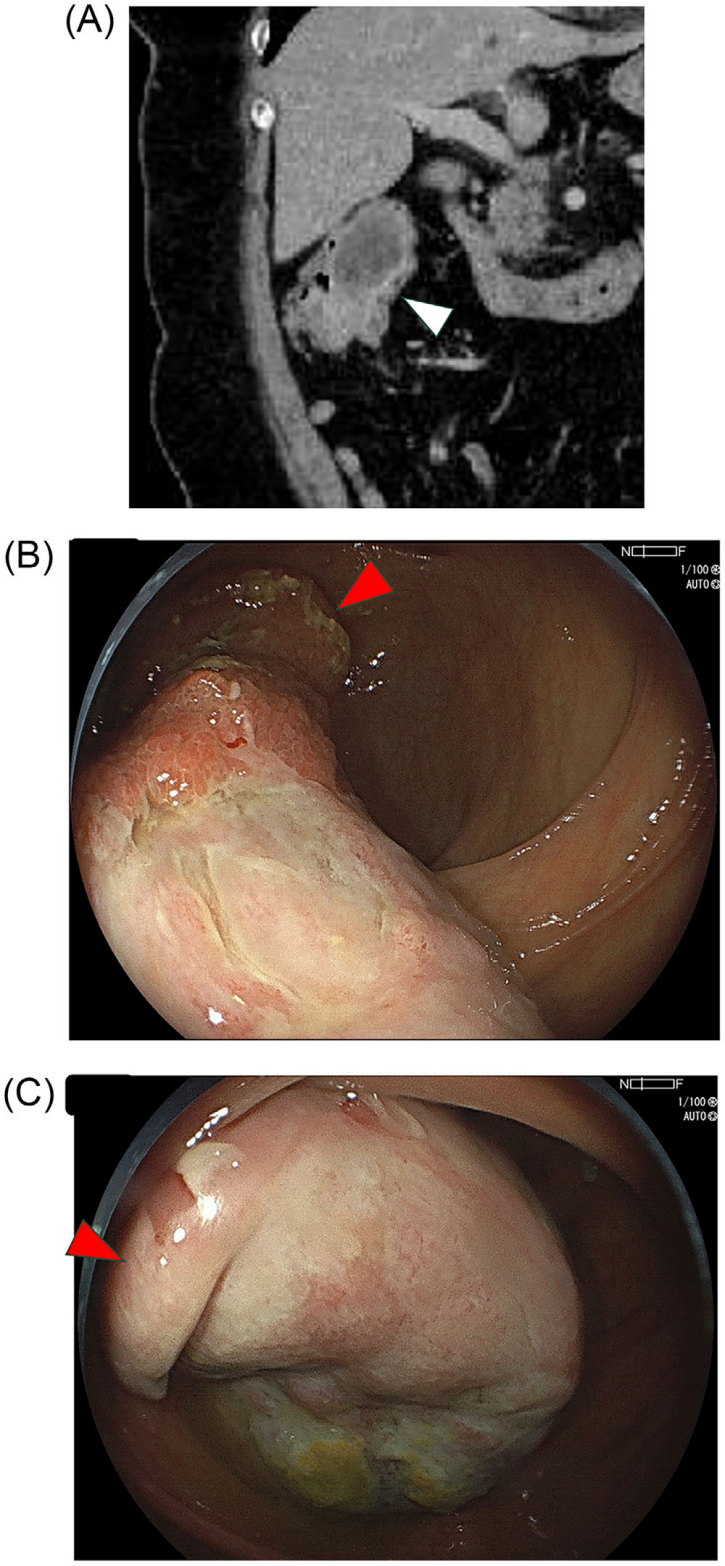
(A) Coronal computed tomography (CT) image. A low‐density lesion with an irregularly enhancing capsule (arrowhead) is visible in the right abdomen, connected to the ascending colon and terminal ileum. (B) Red arrowheads indicate the ileocecal valve. A giant ileal lesion could not be visualized in its entirety at a single glance. (C) Red arrowheads indicate the ileocecal valve. The lesion occasionally migrated into the terminal ileum. Its head was covered with a rough surface, inconsistent with a subepithelial lesion.
